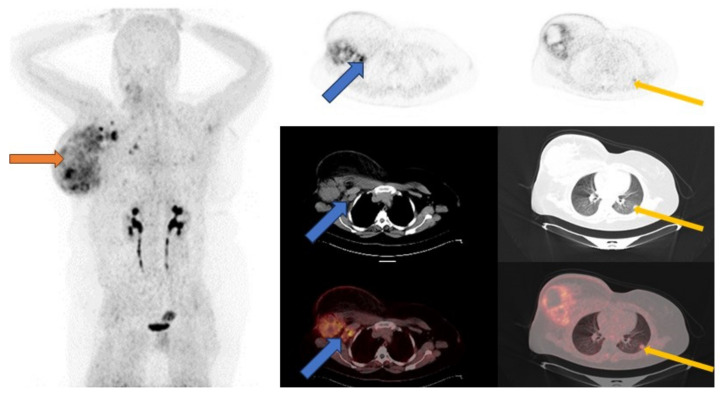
A 39-year-old female referred with locally advanced right breast cancer (TNBC). [^68^Ga]Ga-FAPi-04 PET/CT was performed. Maximum intensity projection (MIP) A: physiological urinary excretion of tracer. Non-benign uptake is also noted on the MIP. This localizes to the enlarged right breast mass (orange arrows), lymph nodes (blue arrows) and left lung (yellow arrows).
